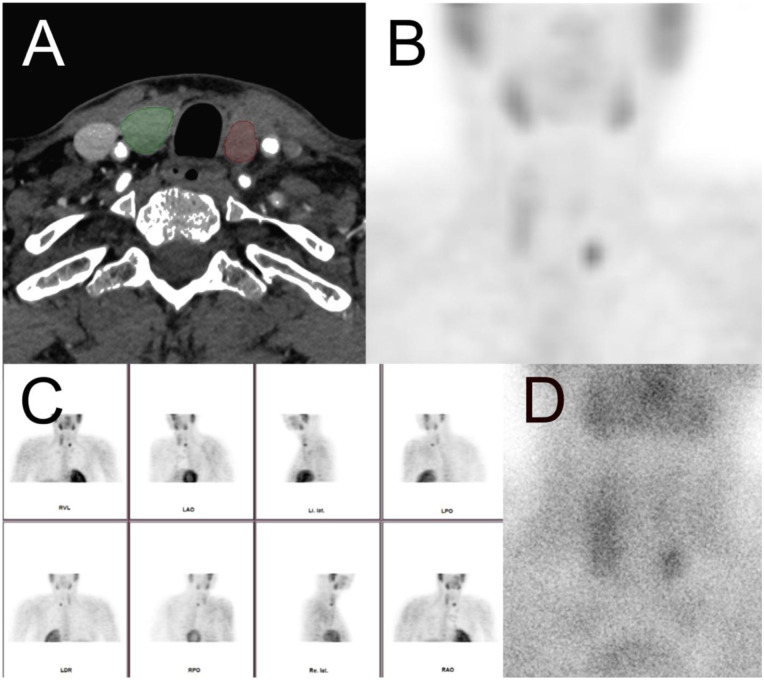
Imaging findings in the patient with primary hyperparathyroidism due to a parathyroid adenoma. (a) Axial contrast-enhanced Computed Tomography (CT) scan of the neck demonstrates a well-circumscribed, hypodense lesion posterior to the left thyroid lobe (marked in red), consistent with a parathyroid adenoma. The right thyroid lobe is shown for comparison (green). (b) Coronal PET image showing increased tracer uptake at the site of the left parathyroid adenoma, indicating metabolic activity. (c) Multiplanar scintigraphy images using a Sestamibi scan demonstrate focal tracer retention corresponding to the left inferior parathyroid gland, consistent with an adenoma. (d) Planar image from Single-Photon Emission Computed Tomography (SPECT) or early static scintigraphy phase further confirms focal activity in the left parathyroid region, supporting the diagnosis of a metabolically active parathyroid adenoma. These imaging findings are typical for primary hyperparathyroidism due to a functioning parathyroid adenoma.
